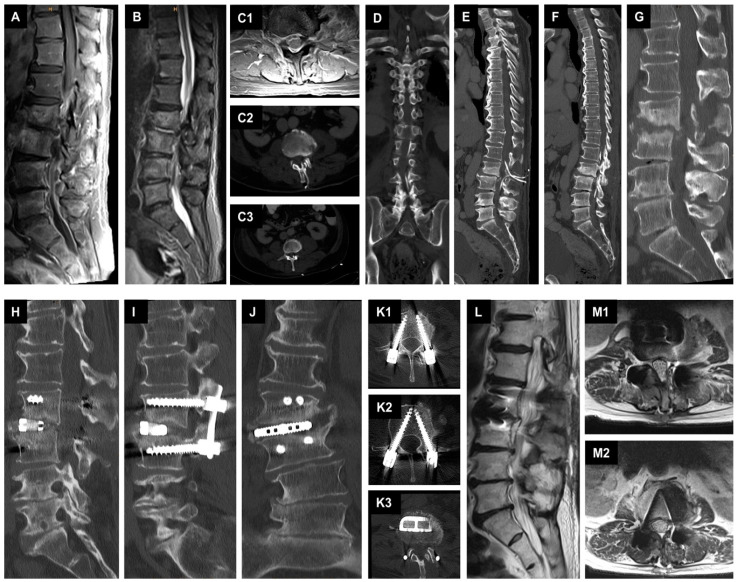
Management of SD with epidural suction–irrigation drainages. (A): A preoperative sagittal T1-weighted fat-saturated contrast-enhanced MR image of the lumbar spine shows spondylodiscitis at the L2/L3 level with epidural spinal empyema at the level of L2 to L5. (B): A preoperative sagittal T2-weighted MR image of the lumbar spine. (C1): Preoperative axial T1-weighted fat-saturated contrast-enhanced MR image of the level L2/L3. (C2–F): Postoperative axial, coronal, and sagittal reformatted CT images showing the placed epidural suction-irrigation drainages cranially and caudally in the spinal canal at the level of L3/L4. (G): Sagittal reformatted CT image showing bone destruction at the level of L2/L3 at 11 weeks at the time of proposed antibiotic discontinuation. (H–K3): Postoperative sagittal, coronal, and axial reformatted CT images showing the result of the single-stage 360-degree instrumentation in a two position. (L–M2): Sagittal and axial T2-weighted MR images displaying the result after stopping the antibiotics.
